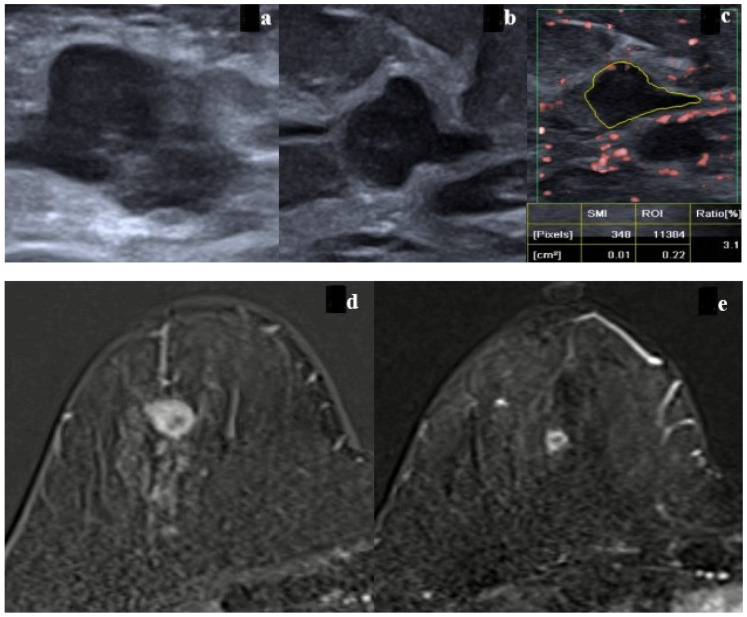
A 44-year-old female patient with an Estrogen Receptor (ER)-negative, Progesterone Receptor (PR)-negative, Human Epidermal Growth Receptor 2 (HER-2) 3+-positive, Ki-67 60%, nuclear-grade-3 malignant breast mass. (a) Pre-neoadjuvant chemotherapy (NAC) ultrasonography shows the mass. (b) Post-NAC ultrasonography (US) demonstrates concentric shrinkage; however, persistent convex mass morphology was interpreted as partial response. (c) Post-NAC Superb Microvascular Imaging (SMI) reveals internal vascularity within the tumor bed (SMI index: 3.1). (d) Pre-NAC contrast-enhanced T1-weighted MRI shows a heterogeneously enhancing mass. (e) Post-NAC Magnetic Resonance Imaging (MRI) demonstrates concentric shrinkage with persistent peripheral enhancement. Although US and MRI suggested partial response, a high SMI index was noted in the tumor bed, confirming a partial pathological response.
